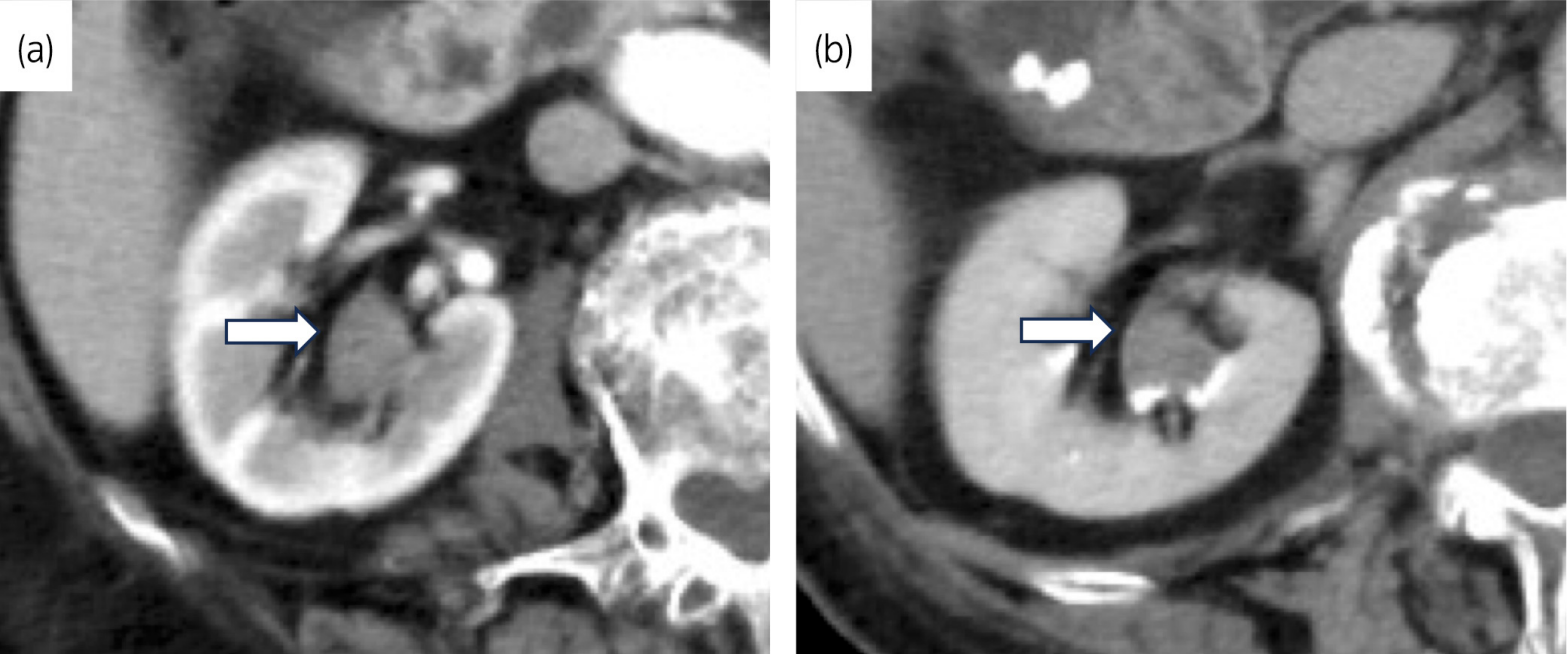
Findings of contrast‐enhanced CT at initial diagnosis of renal pelvic tumor. (a) Arterial phase of contrast enhanced CT. A white arrow shows renal pelvic tumor. (b) Excretory phase of contrast enhanced CT. A white arrow shows renal pelvic tumor.
